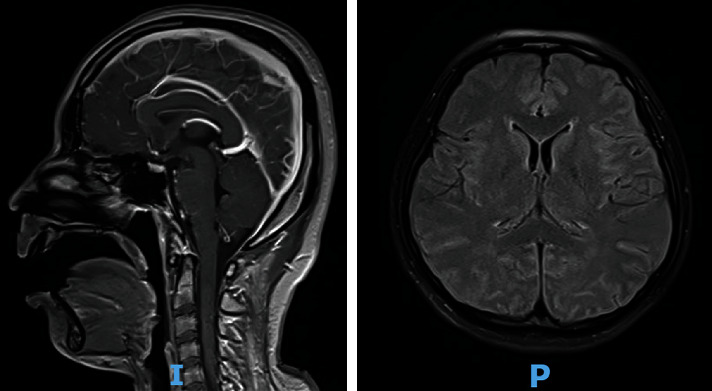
Brain magnetic resonance imaging on the same day of admission to the intensive care unit showed diffuse brain edema and hyperintensity of the bilateral sulcus, gyrus and basal ganglia on fluid-attenuated inversion recovery (FLAIR), and mildly contrast enhancement of the meninge, compatible with hypoxic-ischemic encephalopathy.
